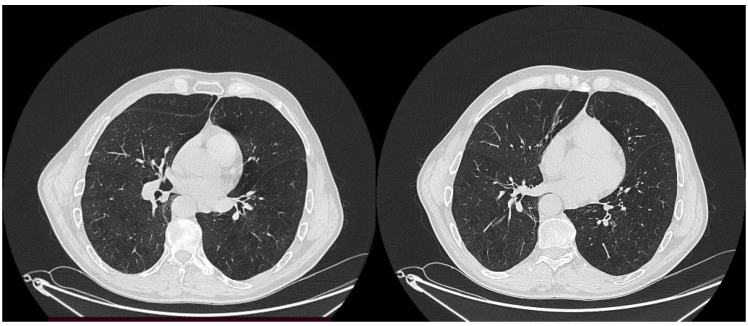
CT scan (2019) before COVID-19 infection, for evaluation of chronic respiratory insufficiency—diffuse lung emphysema.
